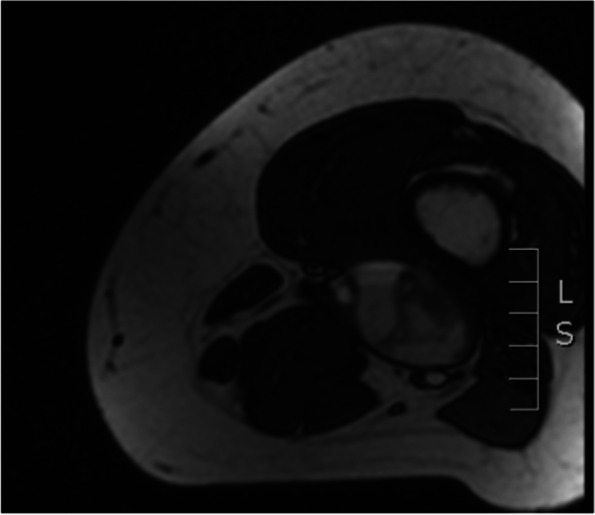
MRI (transverse view) demonstrating thrombus with aneurysm sac, mixed signals consistent with thrombosis of varying ages, and non-occlusive thrombosis within the profunda femoris vein with moderate stenosis
